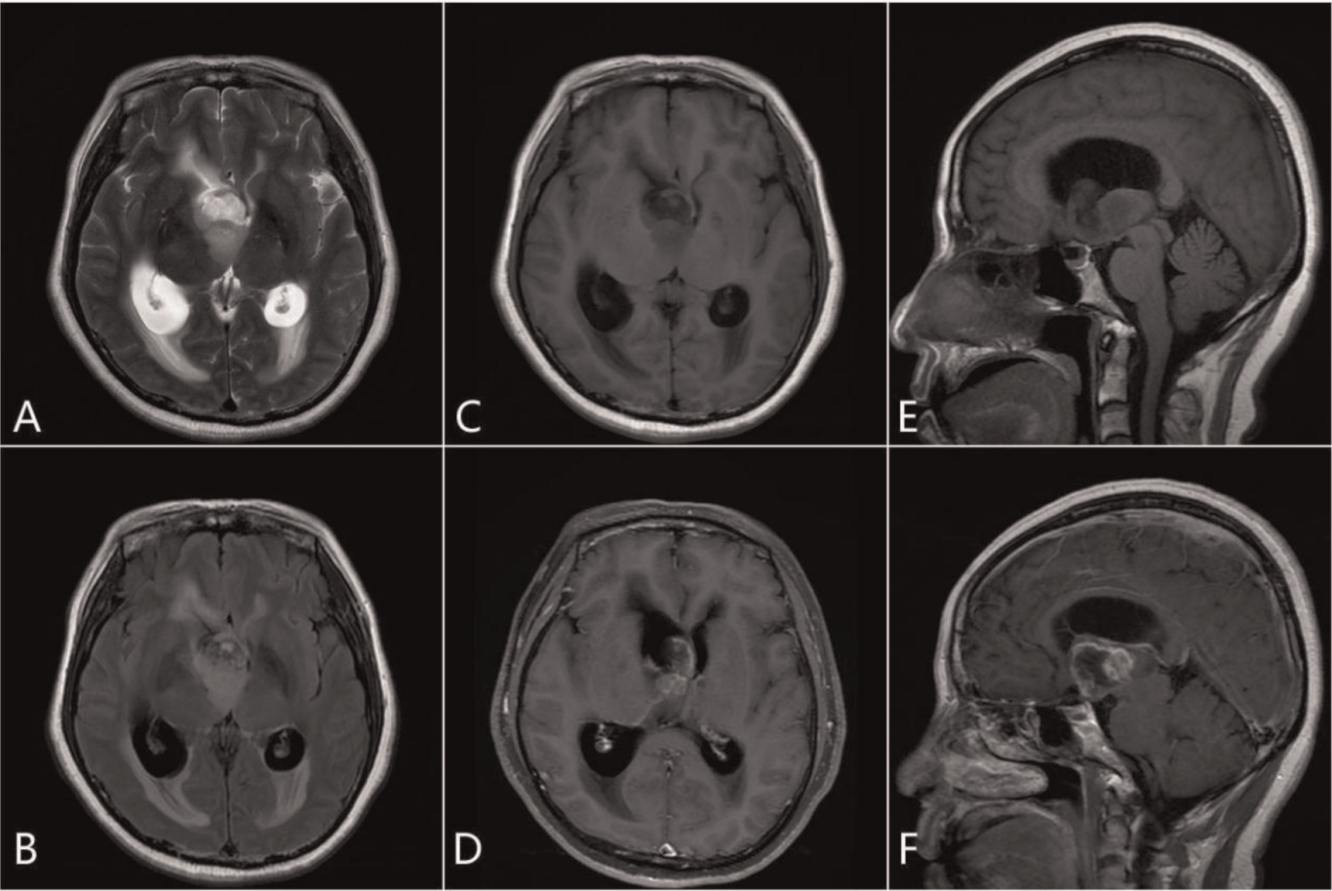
The third ventricle showed isometric T1, slightly longer T2 with mild mixed signal mass shadow, cystic solid. The boundary was clear, with no definite edema signal in the adjacent brain tissue. The main body of the lesion was located in the third ventricle and spread to the right lateral ventricle. Bilateral ventricular dilatation was observed, wherein the right ventricle was more significant. A long stripe T2 signal can be seen at the edge of the lateral ventricle (interstitial brain edema). Enhanced scanning of the mass sac wall and solid components showed irregular ring enhancement, and local lace-like enhancement (A, axial T2; B, axial T2 FLAIR; C, axial T1; D, axial T1 enhancement; E, sagittal T1; F, enhanced sagittal T1).
